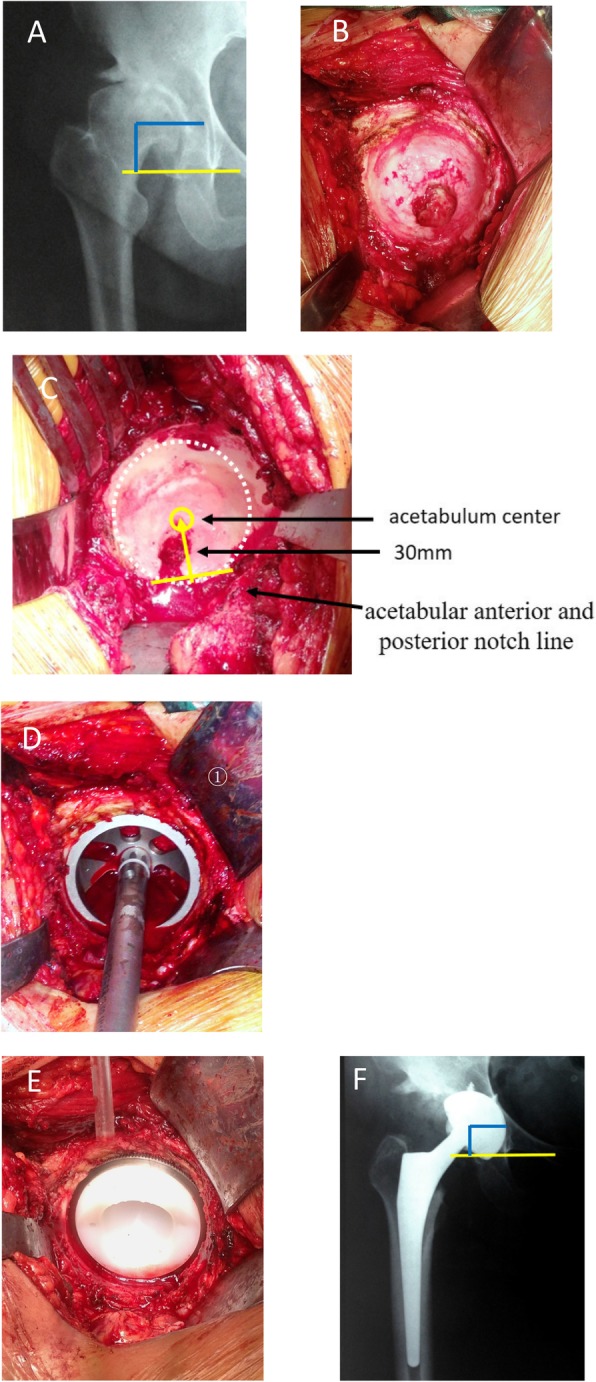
Radiographs of a 39-year-old female patient with end-stage osteoarthritis secondary to Crowe type I DDH. a Preoperative X-ray. b Intraoperative image showed shallow cup-shaped acetabulum and crack-shaped Harris fossa. c Locating the acetabulum center. d Reaming the acetabulum and installing the test cup. e Installing the acetabular prosthesis. f Postoperative X-ray and rotation center restoration
